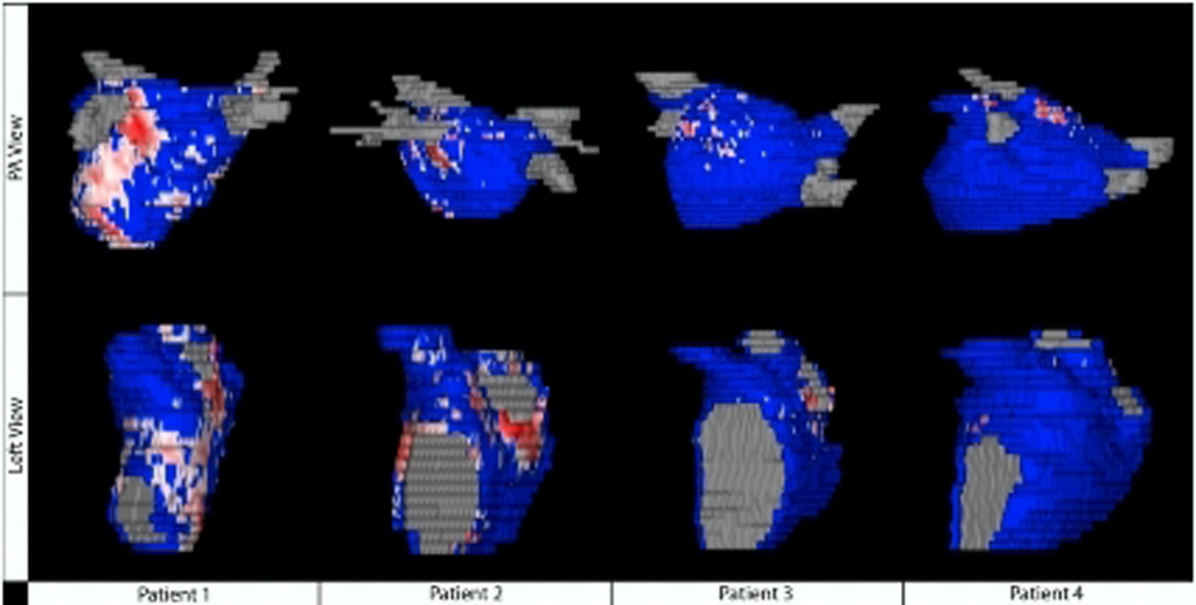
Comparison of late gadolinium enhancement in the left atrium in patients with and without external beam radiotherapy. Posteroanterior (above) and left lateral (below) views of the atrium of LGE -MRI in patients with a history of radiation (patients 1 and 2) and in control patients (patients 3 and 4) demonstrating increased signal with the radiation exposure. Patients 1 and 2 showed enhancement in 17.15% and 7.72% of the left atrium, respectively, while Patients 3 and 4 showed enhancement in 1.80% and 0.87%, respectively, of the left atrium.
